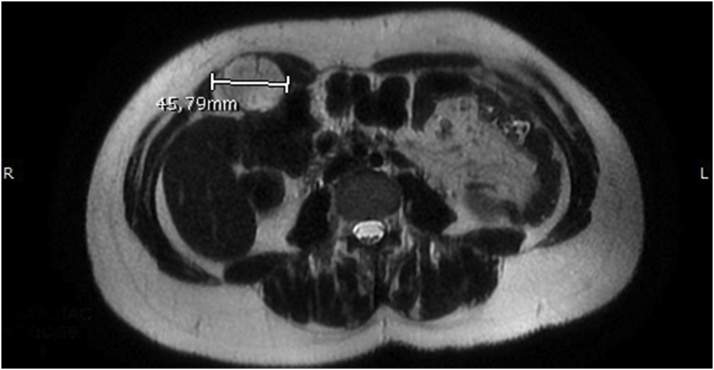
MRI detail of the desmoid tumor in the right rectus abdominis muscle.
